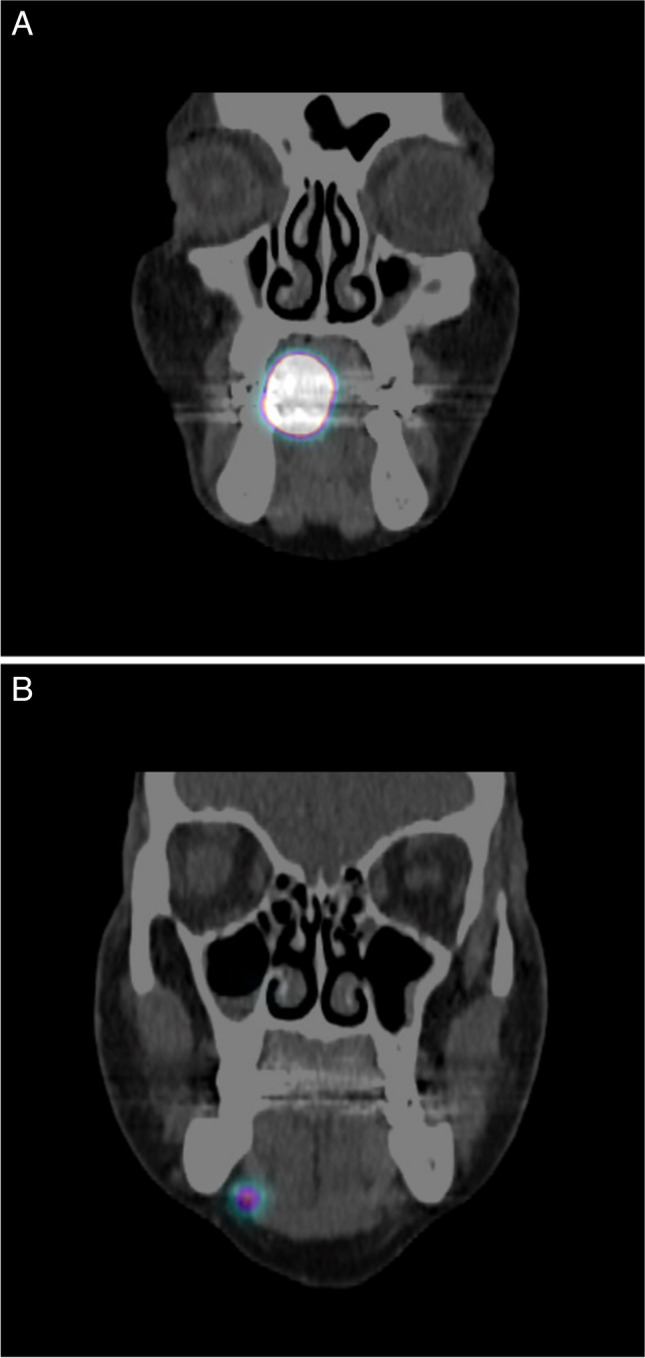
SPECT/CT of patient 5 with visible primary tumor (A) and one SLN (B) after injection of ^99m^Tc radiolabelled human albumin nanocolloid
